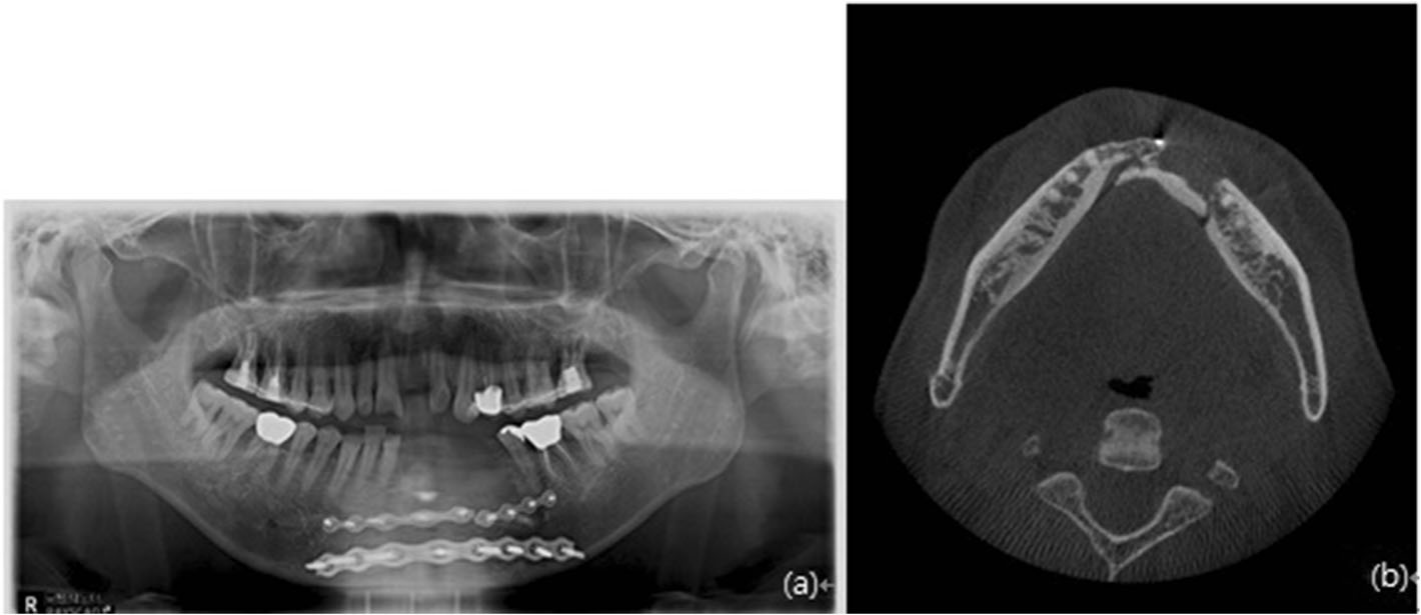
Preoperative radiograph. a Panoramic radiograph and b conical beam computed tomography scan
